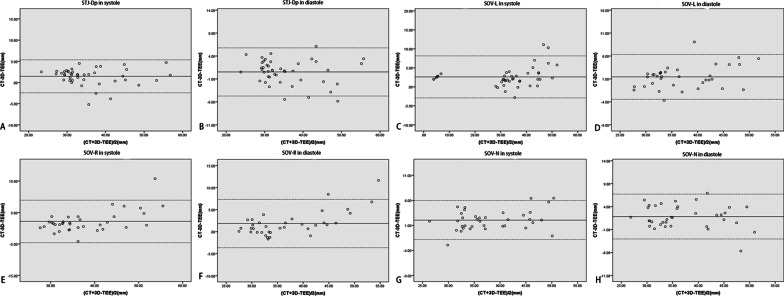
Bland–Altman plot showing good agreement between computed tomography and 3-dimensional transesophageal echocardiography measurements of the sinotubular junction (STJ) and the sinus of Valsalva (SOV). A STJ-perimeter-derived in systole. B STJ-perimeter-derived in diastole. C SOV-left coronary in systole. D SOV-left coronary in diastole. E SOV-right coronary in systole. F SOV-right coronary in diastole. G SOV-noncoronary in systole. H SOV-noncoronary in diastole
